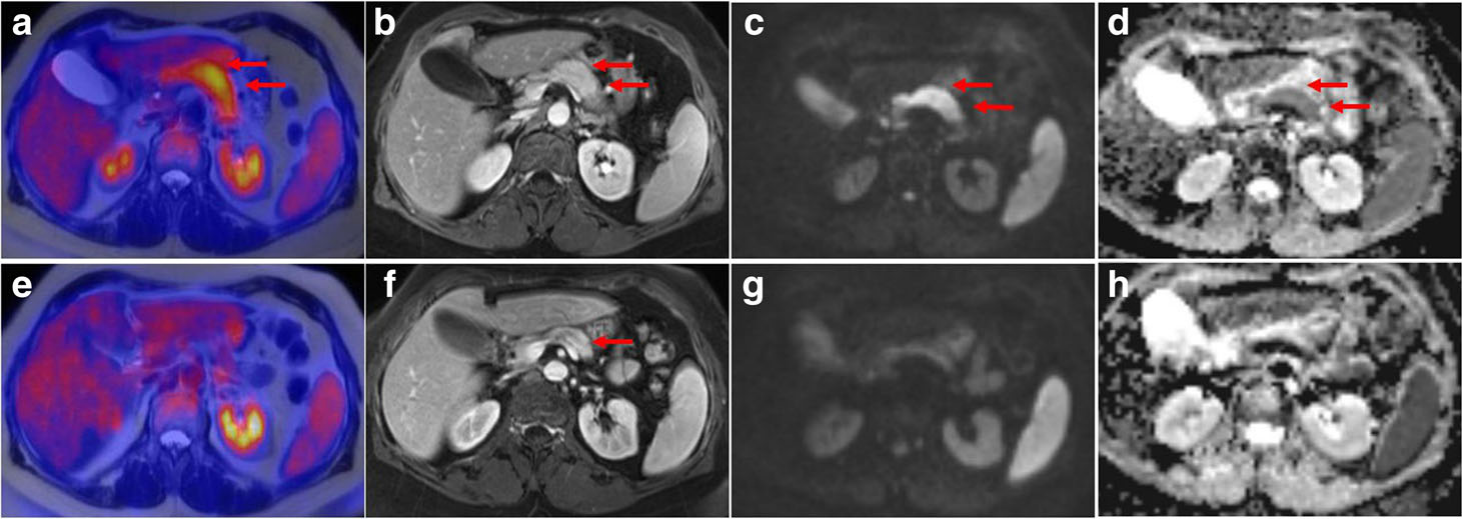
Multiparametric ^18^F-FDG PET/MR in a patient with autoimmune pancreatitis and follow-up ^18^F-FDG PET/MR after initiation of steroid therapy
